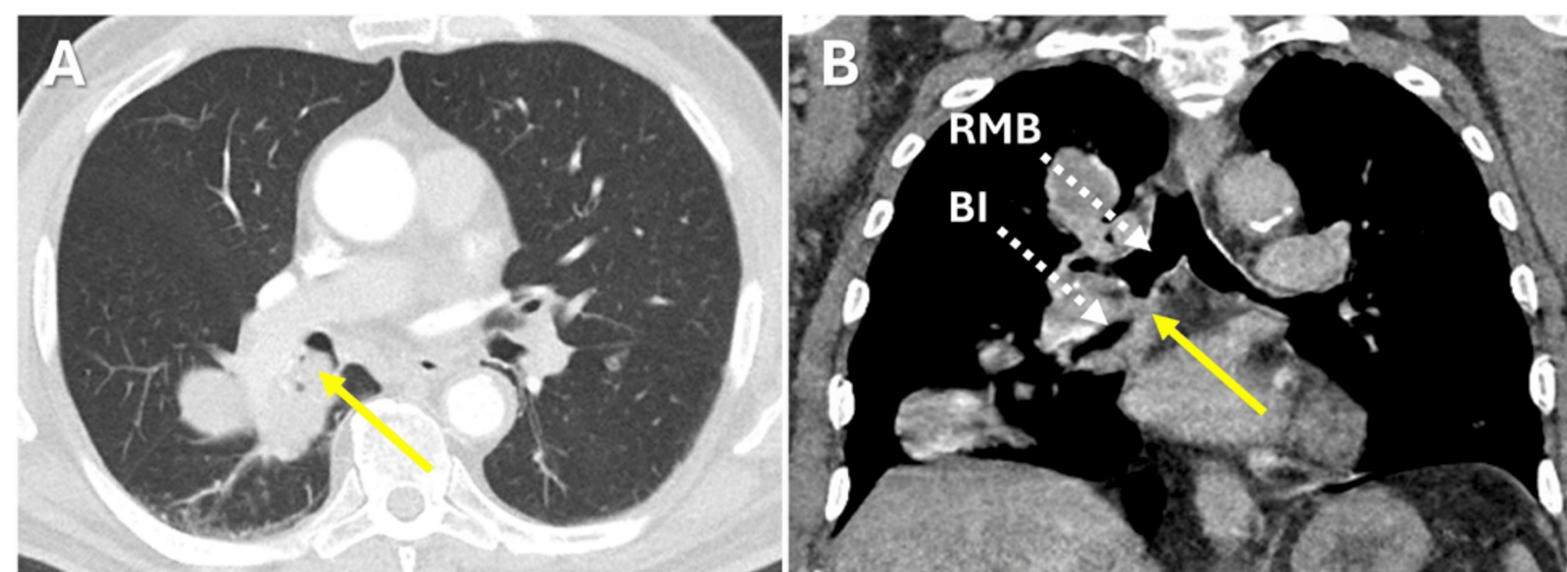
Axial (Panel A) and sagittal (Panel B) CT chest images demonstrating endobronchial metastasis (yellow arrows) causing obstruction between the right mainstem bronchus (RMB) and bronchus intermedius (BI).
